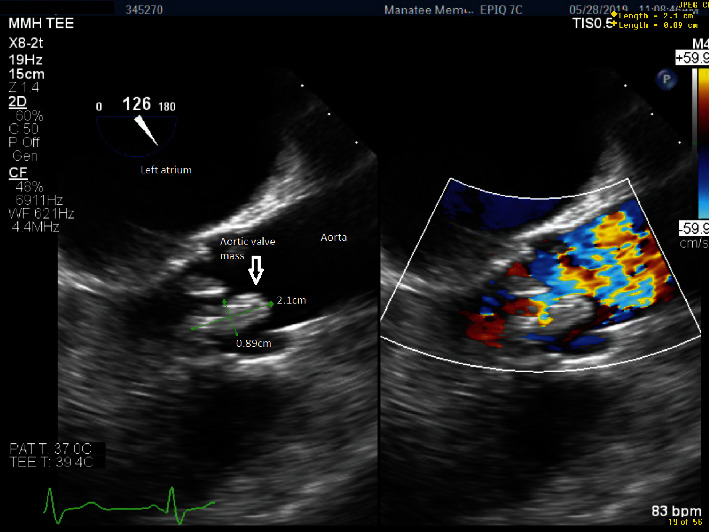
TEE: long-axis view of the aortic valve shows partially calcified aortic leaflet vegetation with significant aortic regurgitation per Doppler study.
